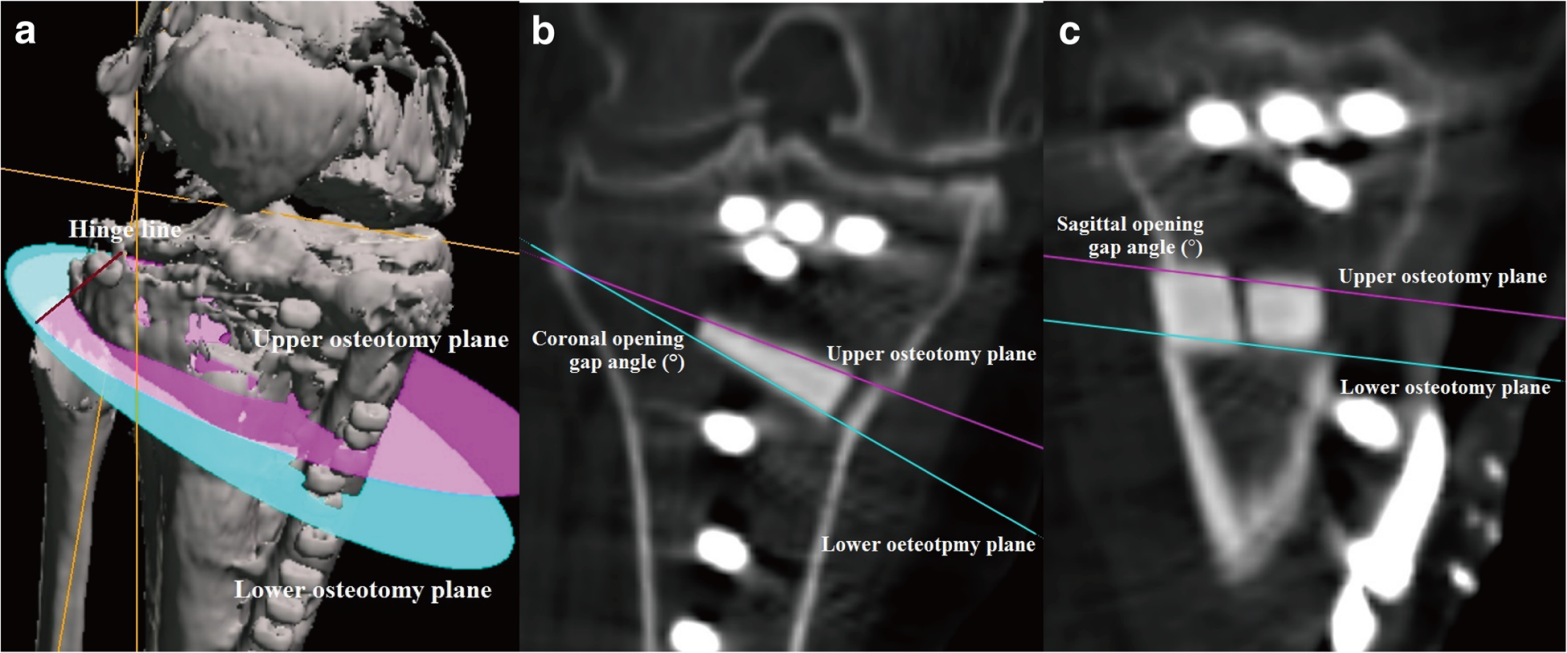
a Three-dimensional computed tomography images of the knee joint. b The coronal opening gap angle between the upper and lower osteotomy planes in the coronal reference plane. c The sagittal opening gap angle between the upper and lower osteotomy planes in the sagittal reference plane
